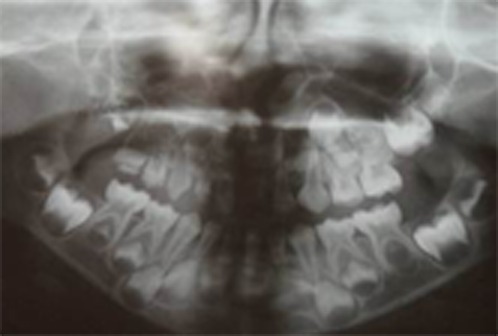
Panoramic radiograph showing the teeth with ghost-like appearance in right maxillary quadrant
